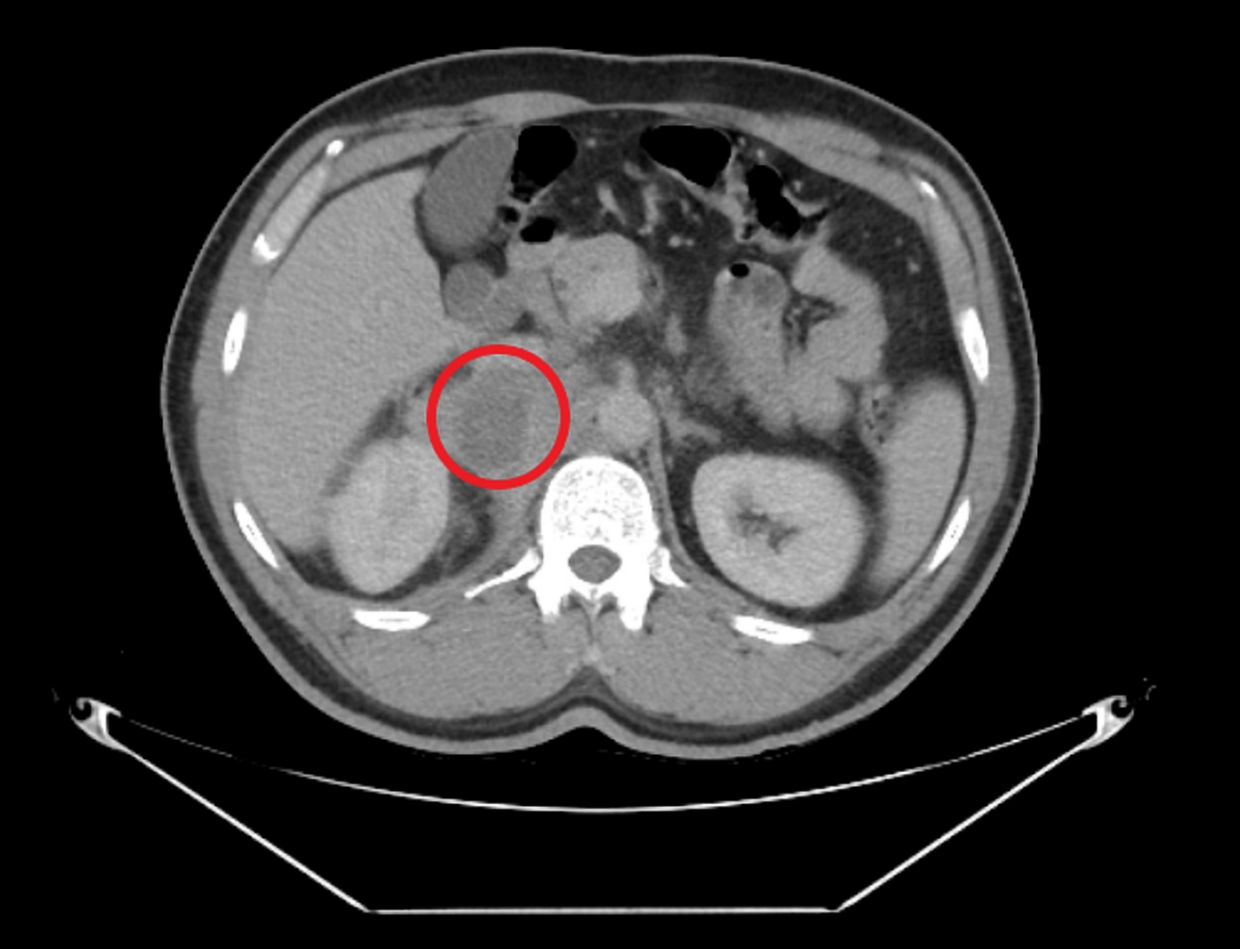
Pelvic computed tomography (CT) scan showing a mass lesion on the right adrenal gland (red circle)
